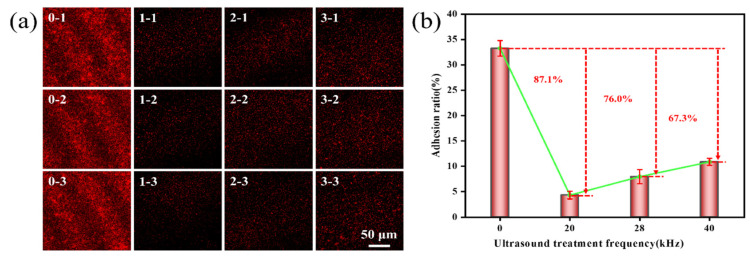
Effect of ultrasonic frequency on adhesion behavior of *Pseudoalteromonas*. (a) CLSM images of *Pseudoalteromonas* under different ultrasonic treatment frequencies: 0–1~0–3(0 kHz); 1–1~1–3 (20 kHz); 2–1~2–3 (28 kHz); 3–1~3–3 (40 kHz). (b) Curve of adhesion rate of *Pseudoalteromonas* with ultrasonic treatment frequency.
